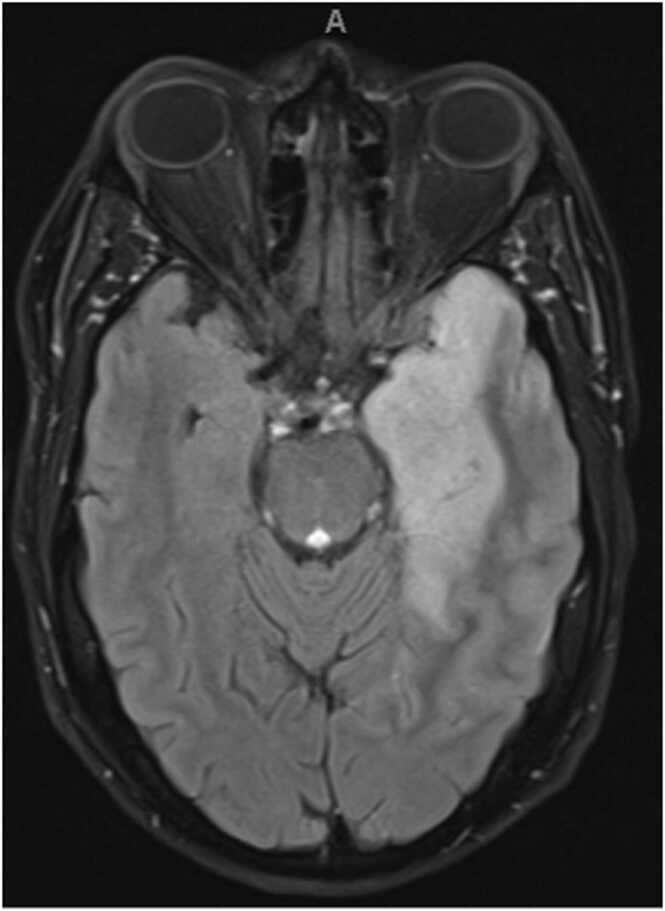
The dynamic contrast-enhanced magnetic resonance imaging (DCE-MRI) of patient’s brain.
